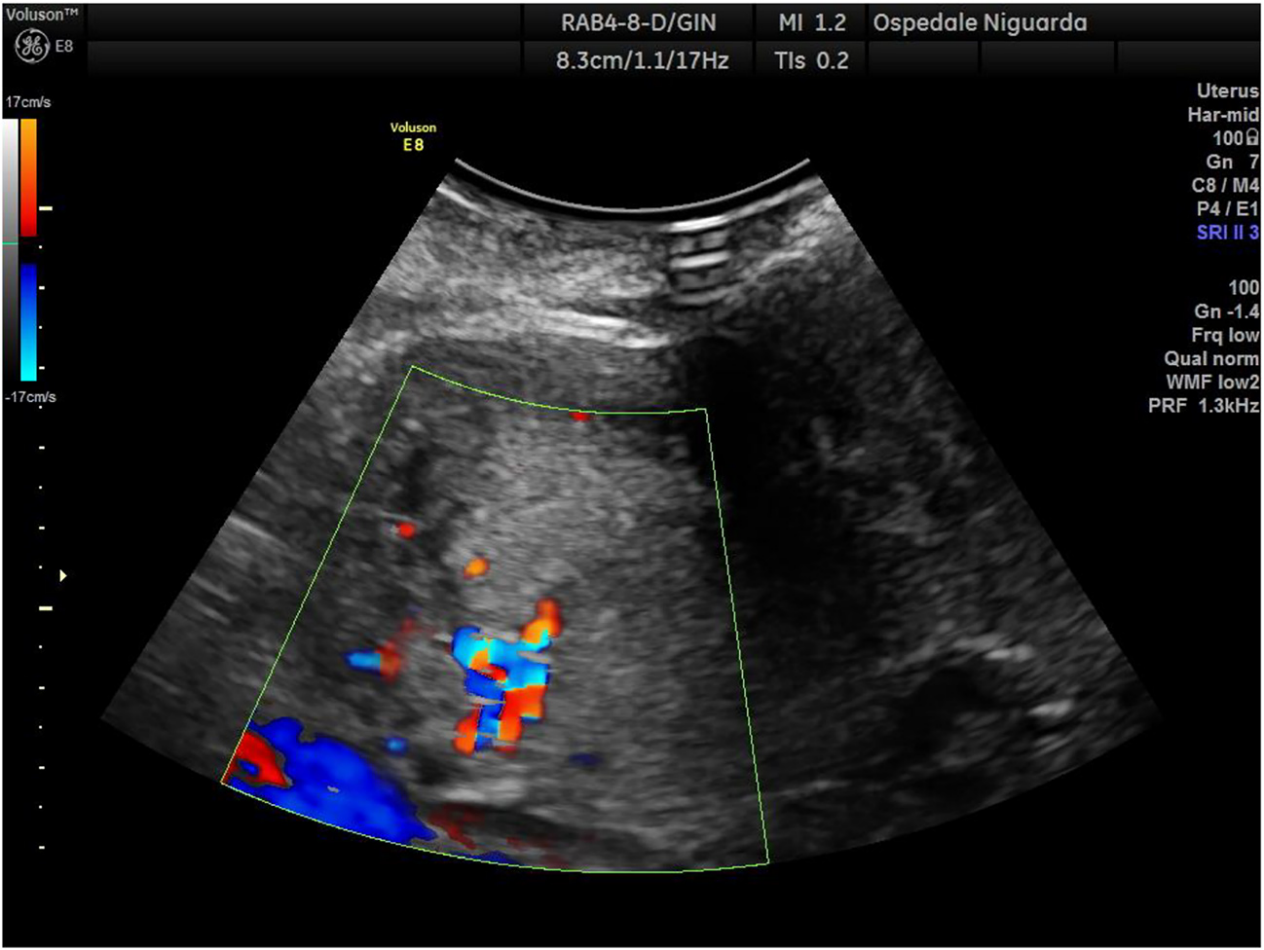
Color-Doppler TVS showing increased vascularization at the placenta-myometrium interface.
